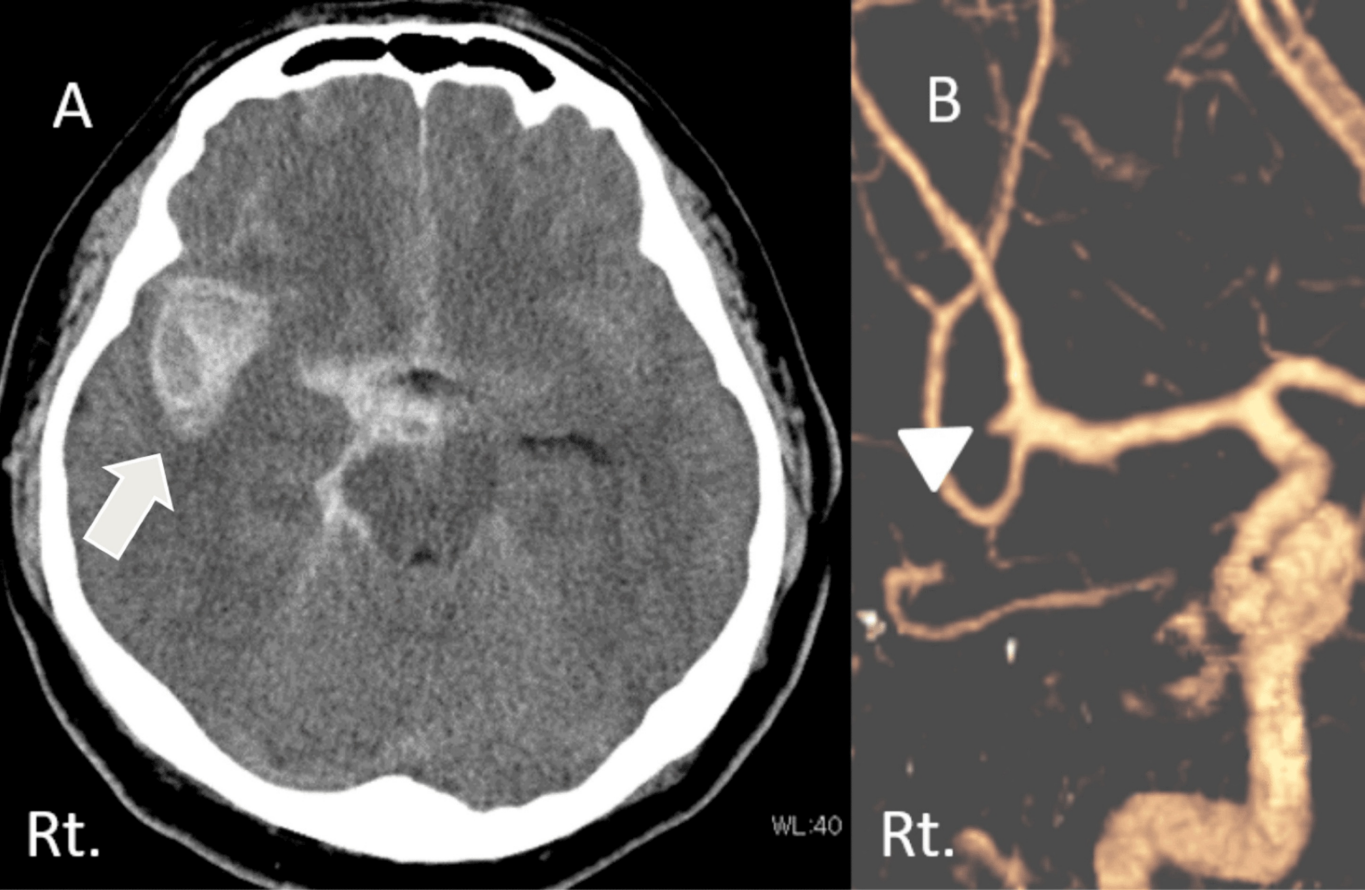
Head CT scan on admission (A) and CT angiography (B) A: Head CT scan showing subarachnoid hemorrhage and right intracerebral hemorrhage (white arrow). B: Contrast CT scan showed a ruptured aneurysm at the bifurcation of the right middle cerebral artery (white arrowhead).
